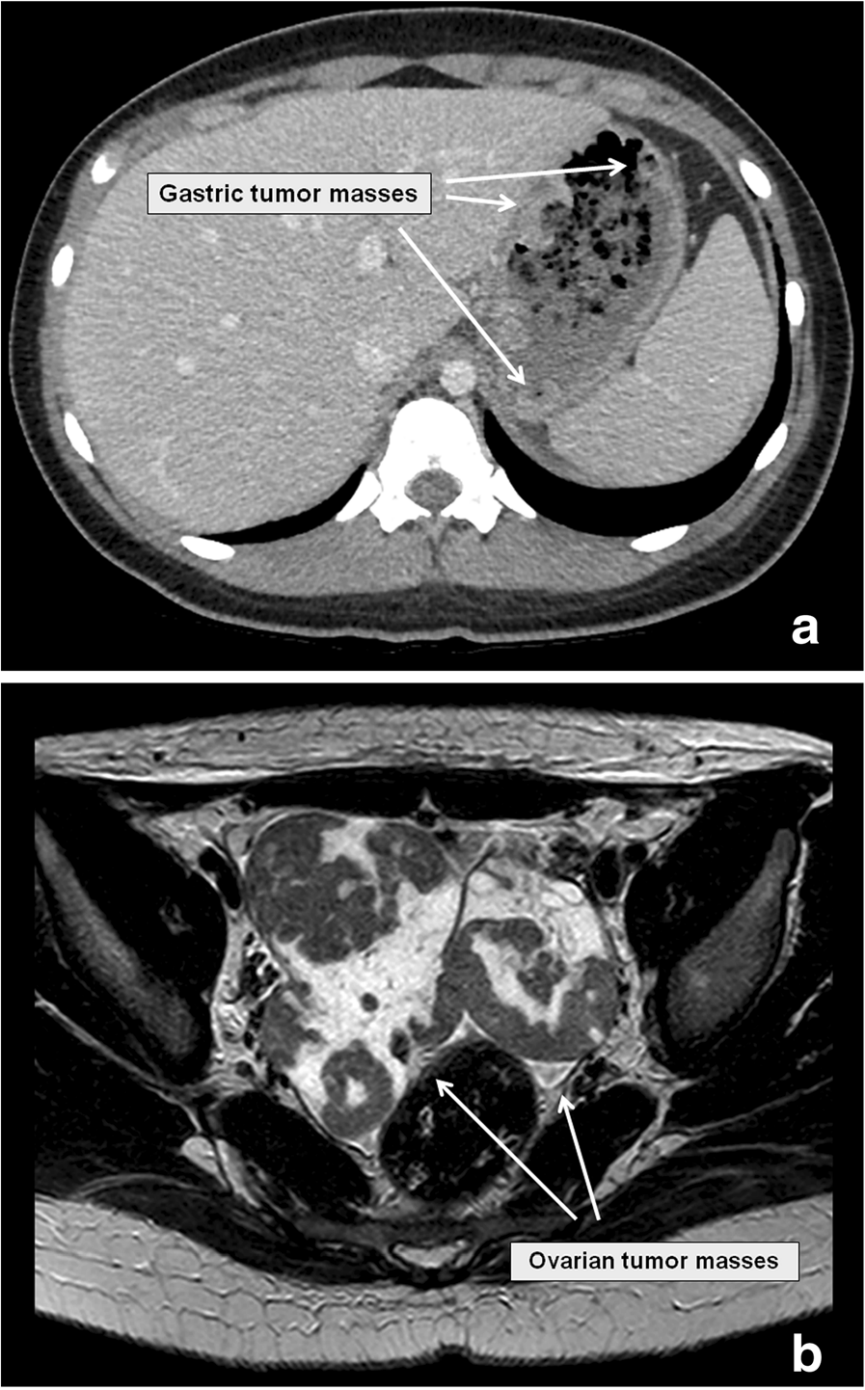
Abdominal CT scan with gastric tumor (a) and MRI showing bilateral ovarian masses (b).
